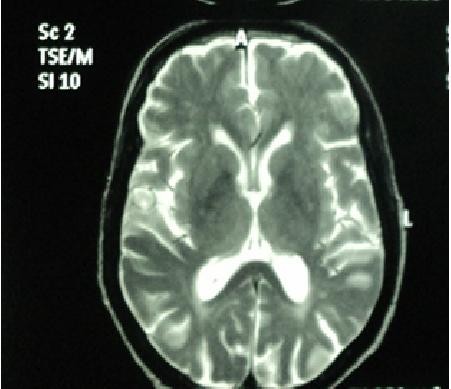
T2 magnetic resonance imaging image showing hyperintensities in periventricular areas.
